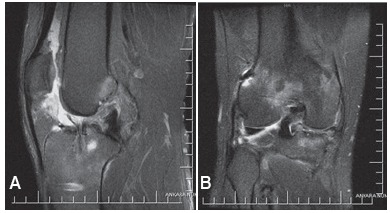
ACL re-rupture was seen 3 months after surgery on the sagittal view seen on MRI (A). The medial meniscus was intact and healed completely (B).
